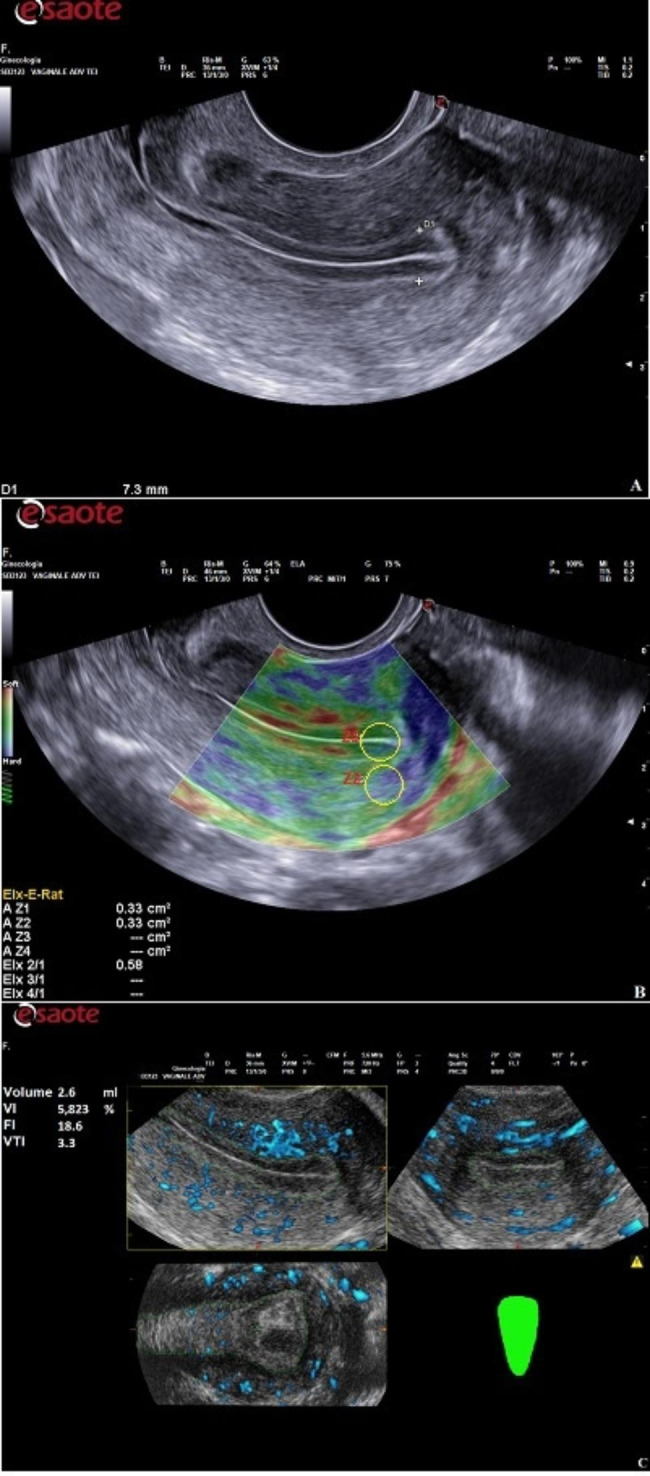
The ultrasound examination protocol: (A) B mode US shows a normal anteverted uterus with endometrial thickness of 7.3 mm. (B) Endometrial elastosonography and strain ratio (Z2/Z1) calculation in a sagittal plane: endometrium (Z1), adjacent myometrium (Z2). The strain rate (SR) is calculated automatically by the software. SR = 0.58. (C) Endometrial Volume (EV) and 3D Power Doppler vascularity indices are calculated automatically after a manually reconstruction using the Virtual Organ Computer-aided Analysis (VOCAL) software (XVRA ™, Esaote, Italy). Endometrial volume = 2.6ml, VI = 5.823%, FI = 18.6, VFI = 3.3
